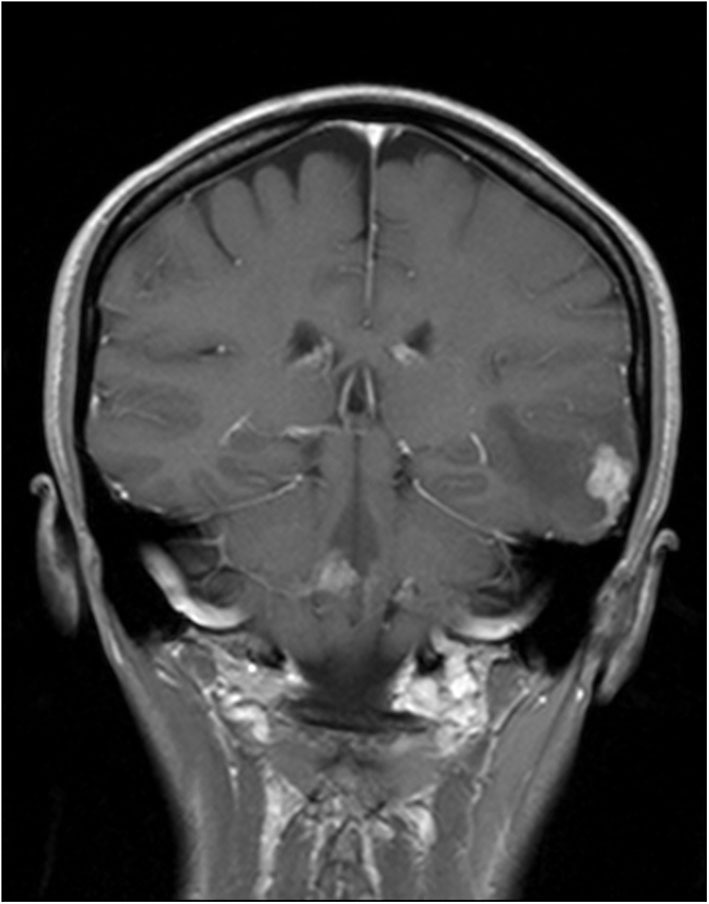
Tumor in the lateral part of the left temporal lobe and the cerebellum in magnetic resonance imaging (MRI) scans.
